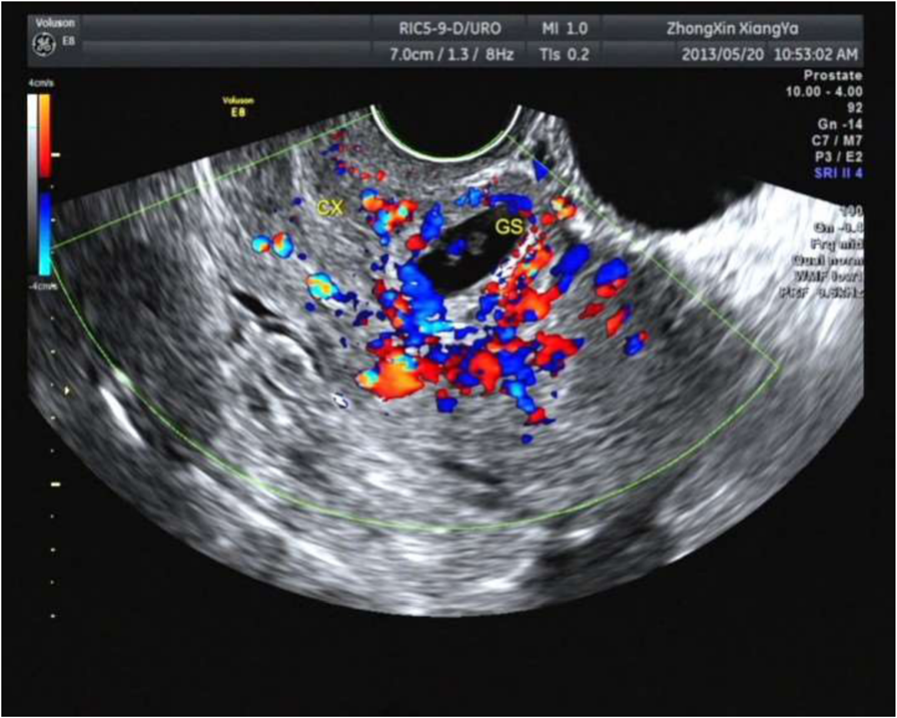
Ultrasound findings of a typical CSP. Longitudinal section of the uterus showing a 6 + 3 weeks with cardiac activity gestational sac (Case 1; crown–rump length:3.6 mm) implanted into a previous Cesarean section scar and protruding towards the urinary bladder with strong peripheral color doppler signals
